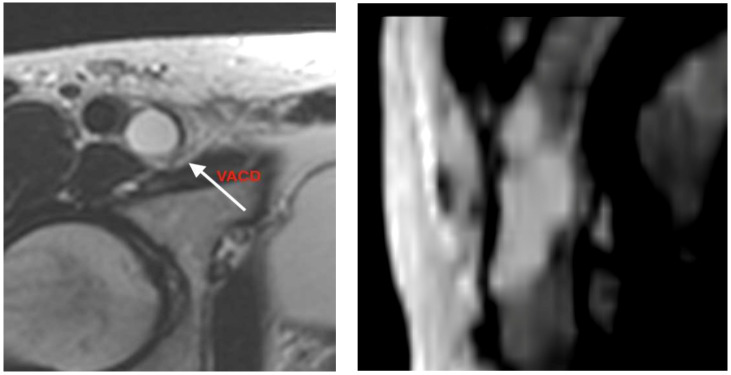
Axial magnetic resonance imaging demonstrating venous adventitial cystic disease, with low signal intensity on T1-weighted images and high signal intensity on T2-weighted images, consistent with mucinous content. The irregular homogeneous structure fills almost the entire lumen. The arrow indicates the cystic irregular lesion.
